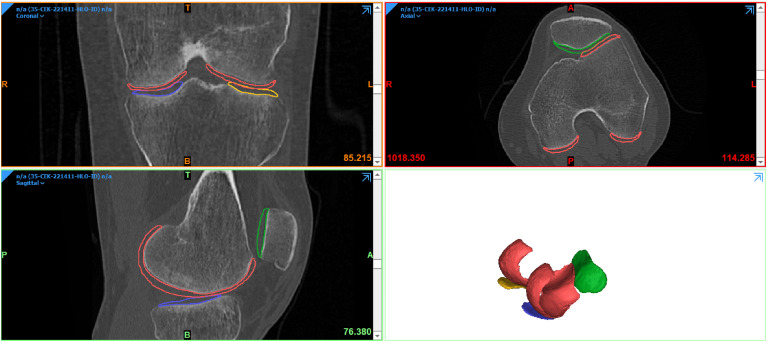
Coronal, axial, and sagittal view of segmented femoral (red), tibial (blue and yellow) and patellar (green) cartilages registered onto CT and corresponding 3D model.
